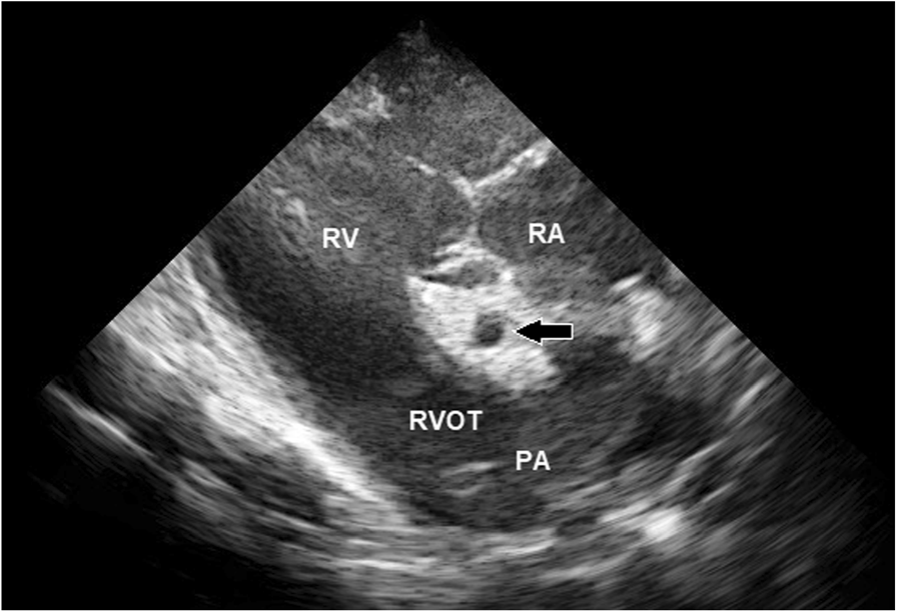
Right parasternal long axis view in 2D ultrasound image of the RCA. The RCA is visible in the middle of the image (white arrow). The right atrium (RA) lies dorsal to the RCA, the right ventricular outflow track (RVOT) is visible lateroventrally to the RCA, the pulmonary artery (PA) is visible ventrally to the RCA while the right ventricle (RV) lies lateral to the RCA
